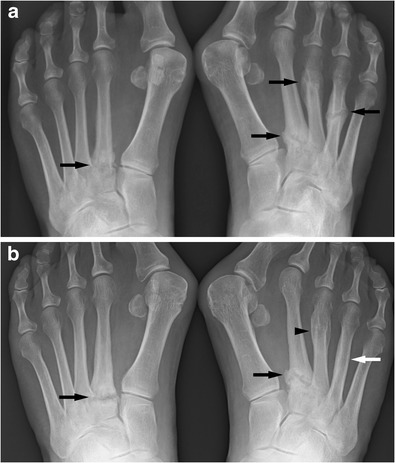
PA radiographs of the feet of a middle-aged female patient. The first radiograph (a) shows stress fractures in both feet in various stages of healing (black arrows). Symmetrical fractures at the base of the second metatarsals. Two years later (b) the fractures at the base to the second metatarsals have still not healed (black arrows); the fracture of the right fourth metatarsal has remodeled well and is now hard to appreciate (white arrow); the fracture of the right third metatarsal has healed (black arrowhead). Stress fractures are not rarely bilateral and symmetrical. Fractures at the base of a metatarsal heal less well than midshaft fractures
